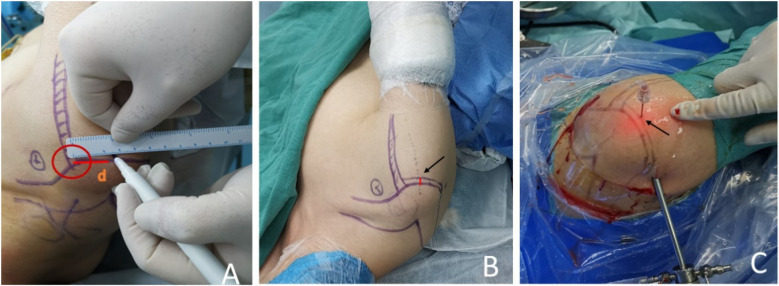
(A) The surgical approach was plotted preoperatively according to the precise positioning technology assisted by three-dimensional CT. (B) Adding the displacement distance of the humeral head caused by traction to the first position, the final position was determined. The arrow shows the distance where the humeral head moves down from the first position. (C) On the final position, we pierced the skin with the needle vertically. The arrow indicates the puncture point.
